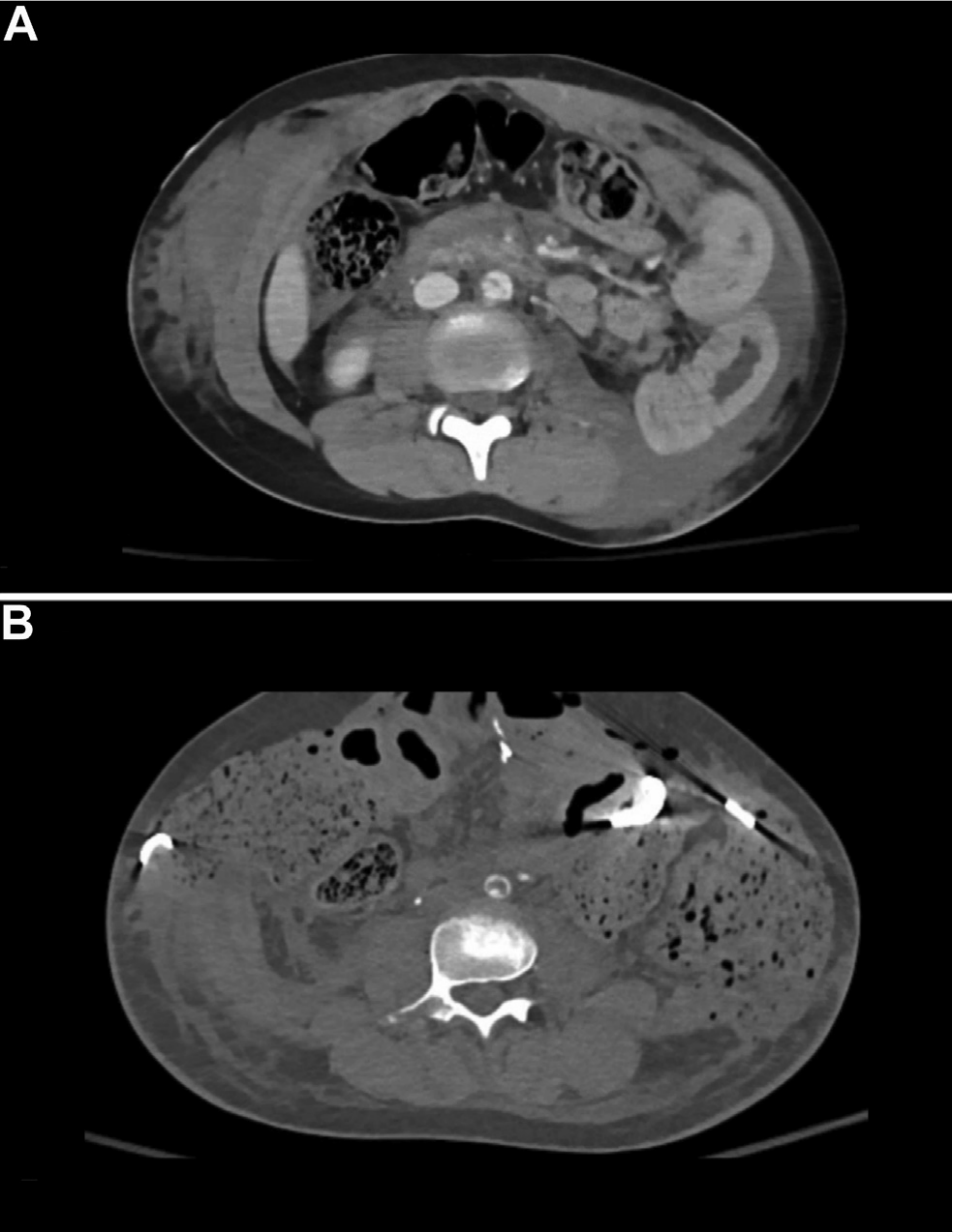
A, Initial computed tomography (CT) angiogram after the injury showing focal aortic dissection. B, Subsequent CT angiogram showing evolution of the aortic injury to worsening dissection and near occlusion of the aorta.
